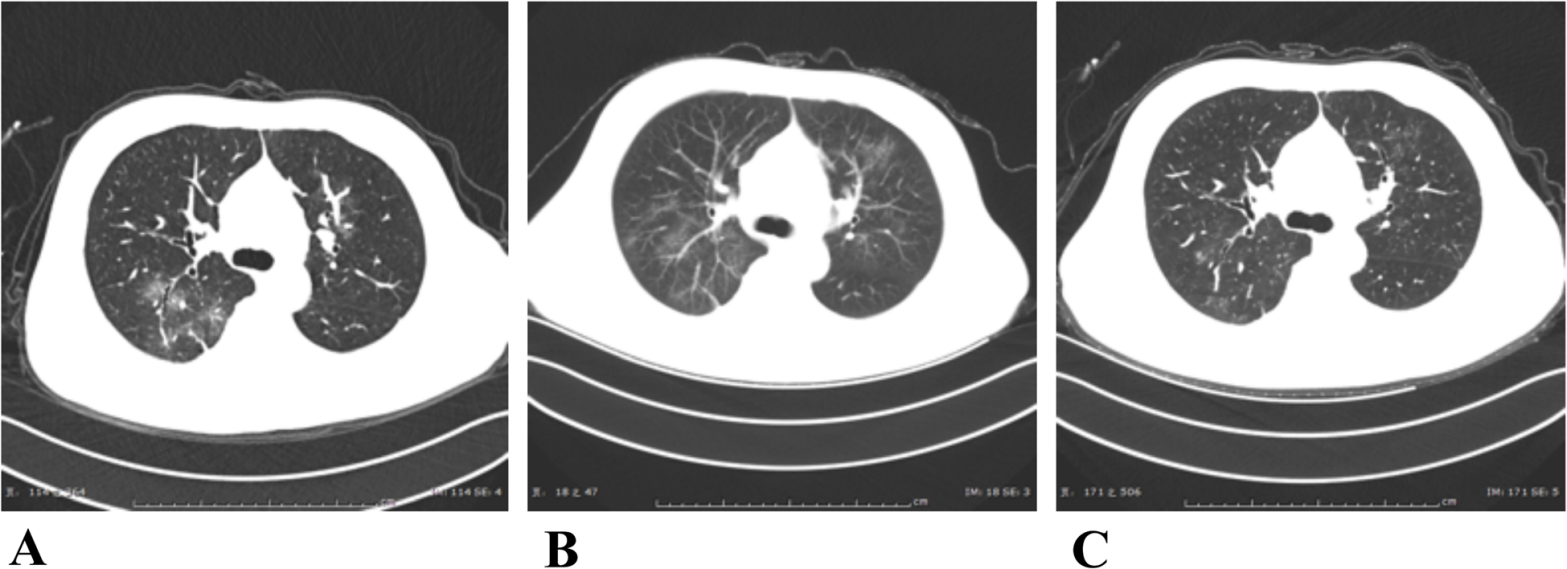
The chest computed tomography (CT) scan showed multiple patchy ground-glass opacities in superior lobe of bilateral lung and inferior lobar of left lung on admission (a), aggravation (increased area of patchy ground-glass opacities) on day 4 (b), and significant improvement of bilateral ground-glass opacities on day 9 (c)
